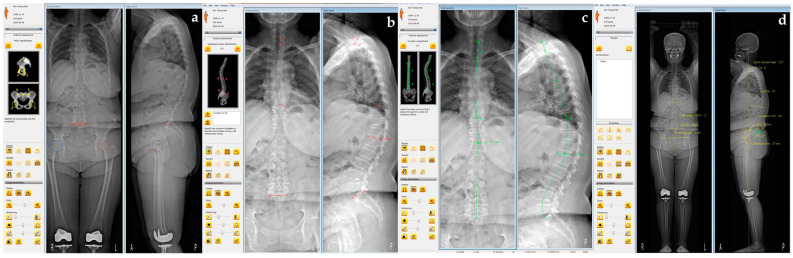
Three-dimensional reconstruction of images using SterEOS^®^ software: (a) After standardizing the radiograph to align the femoral heads in the lateral view, the positions of the T1 and S1 superior endplates are identified. (b) The software generates a line that can be manually adjusted according to the spinal alignment and the center of the vertebral column. (c) Each vertebra’s morphology is defined by identifying 28 points. (d) Finally, a 3D spine model is reconstructed using a database of vertebral 3D morphology.
